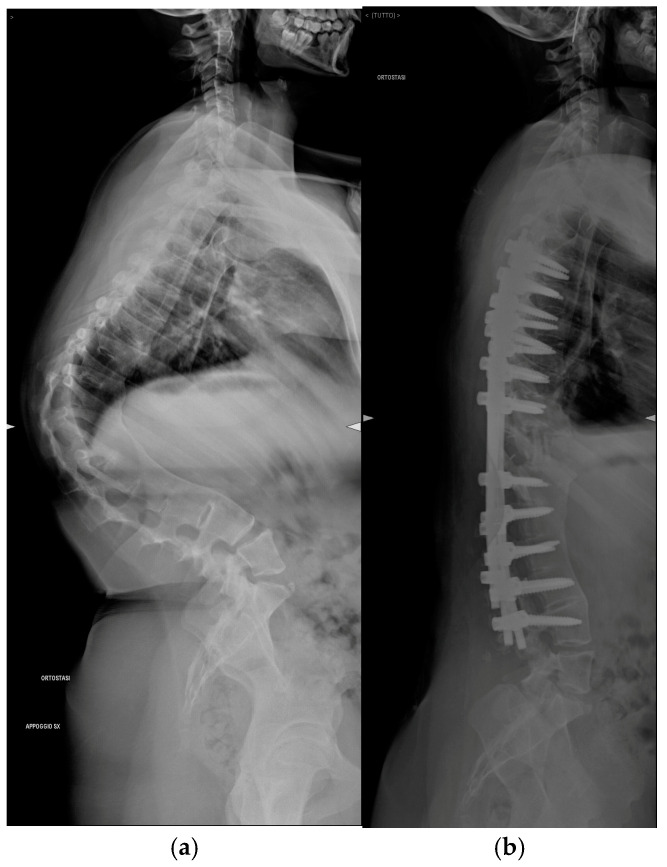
Lateral radiograph of Patient 5. (a) Preoperative assessment. (b) Postoperative assessment.
